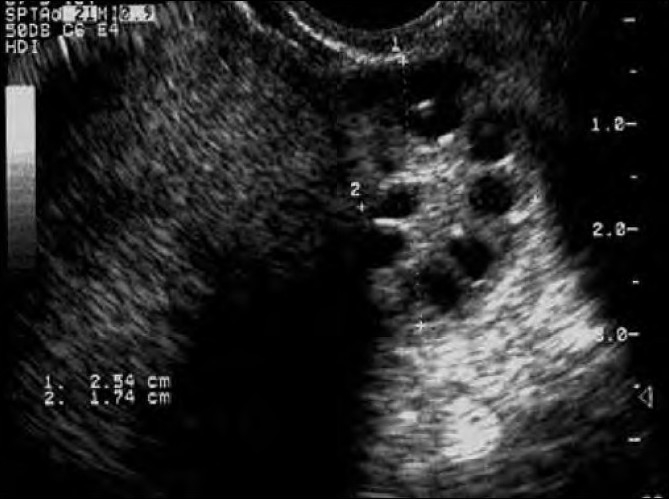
Sonographic appearance of polycystic ovaries
